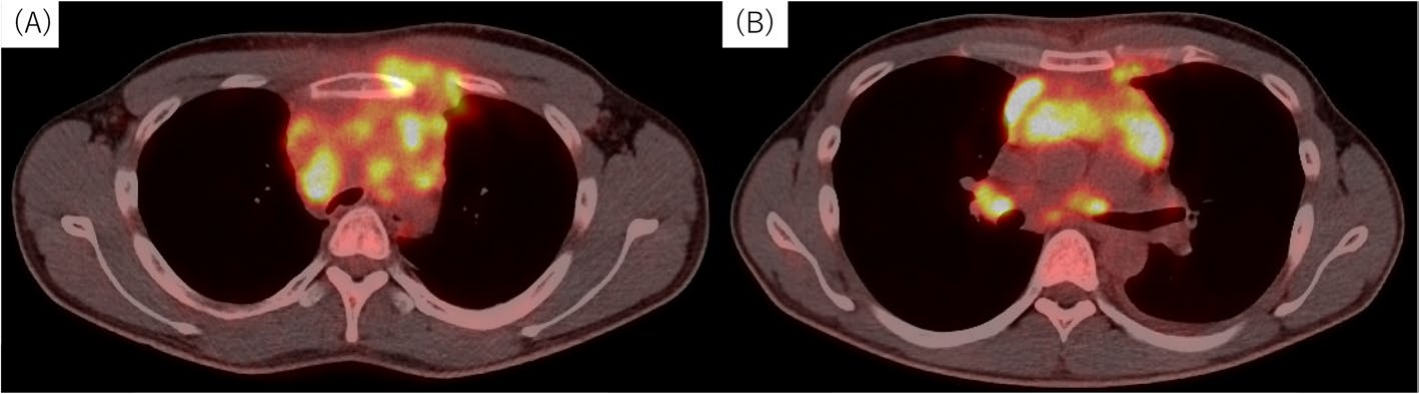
Positron emission tomography-computed tomography image showing high accumulation of the main tumor with direct invasion of the left anterior chest wall (A), and multiple enlarged lymph nodes (B)
